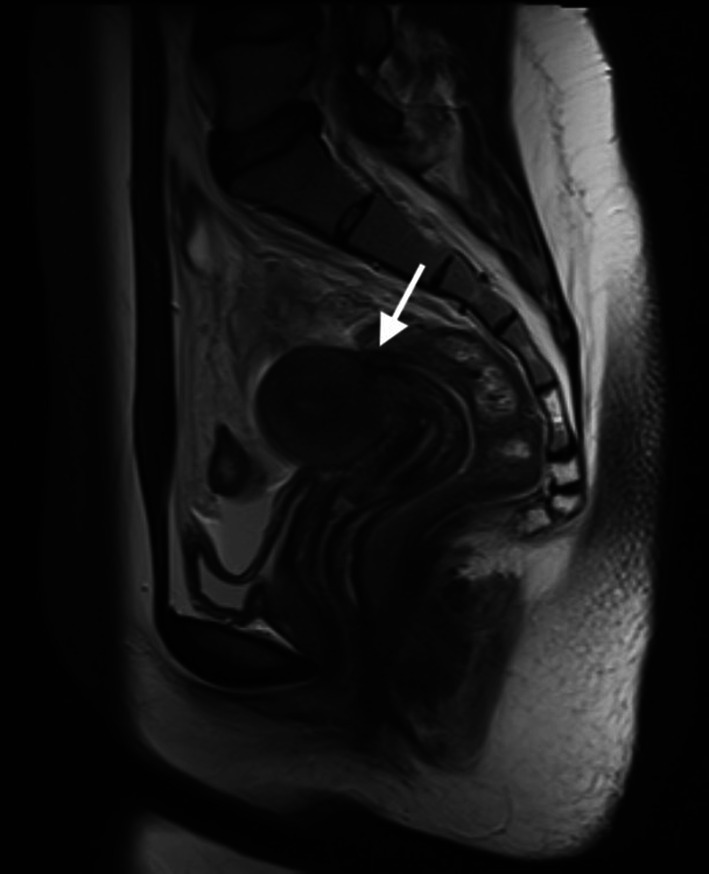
T2 weighted sagittal MRI image showed involvement of Torus-Uterinus as a single site of DIE
